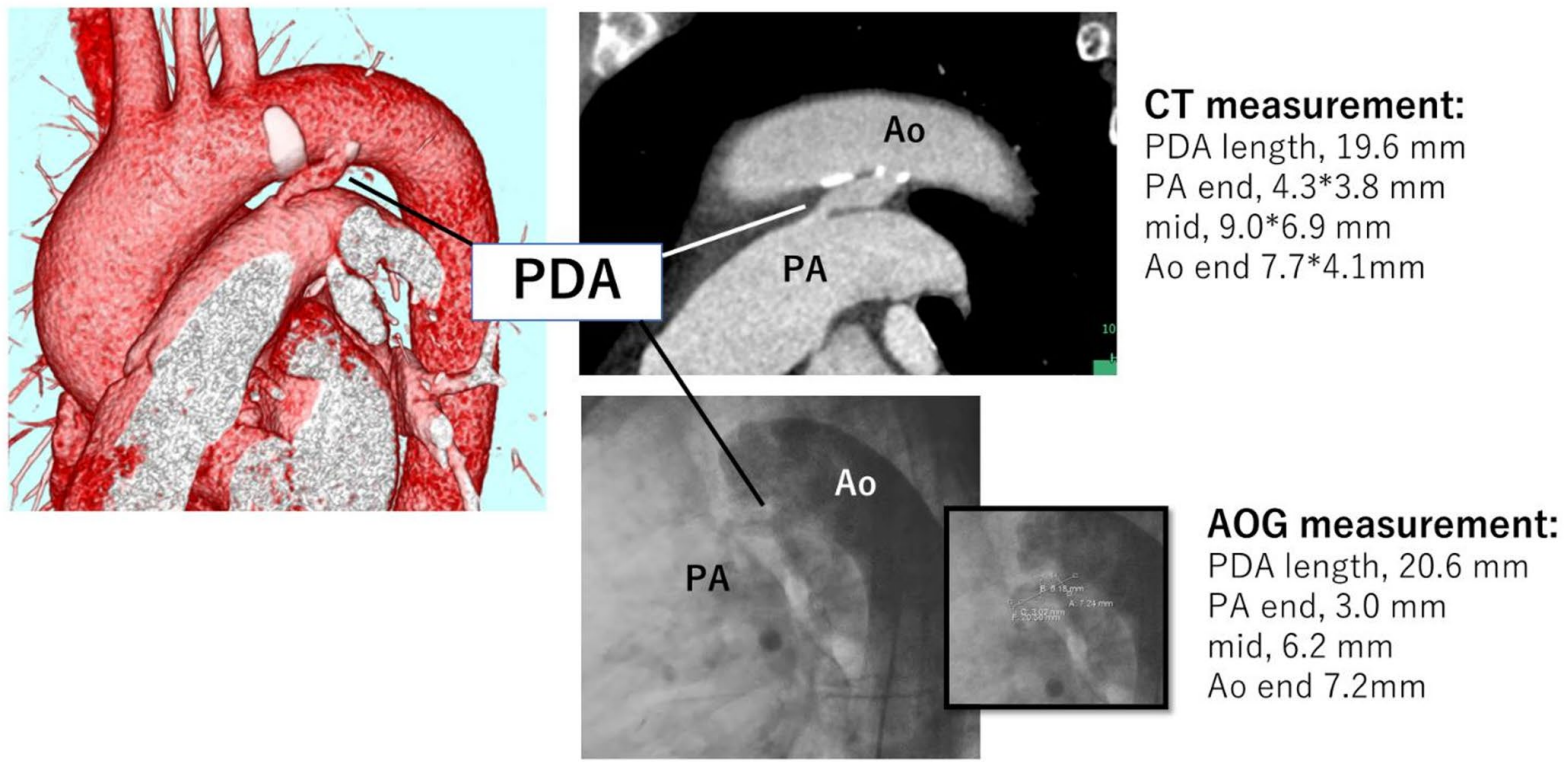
Representative case of PDA assessment using CT and aortography. Preoperative cardiac CT and intraoperative aortography revealed a stretched Krichenko type E PDA. The pulmonary artery end of the PDA, which is the most critical measurement for device selection, was measured larger on CT than on aortography. CT also revealed calcification on the aortic side of the PDA, suggesting the need for caution to avoid vascular injury during device deployment. Left: A three-dimensional volume-rendered CT image showing the PDA connecting the descending aorta and the pulmonary artery. Top right: CT image analysis showed the anatomical course and diameter of the pulmonary artery end (4.3 × 3.8 mm). Bottom right: Aortographic image showing measurements of the PDA at multiple levels: the aortic end (7.2 mm), the pulmonary artery end (3.0 mm), and the middle Sect. (6.2 mm), as well as the length (20.6 mm). PDA, patent ductus arteriosus. AOG, aortography. Ao, Aorta. PA, pulmonary artery
